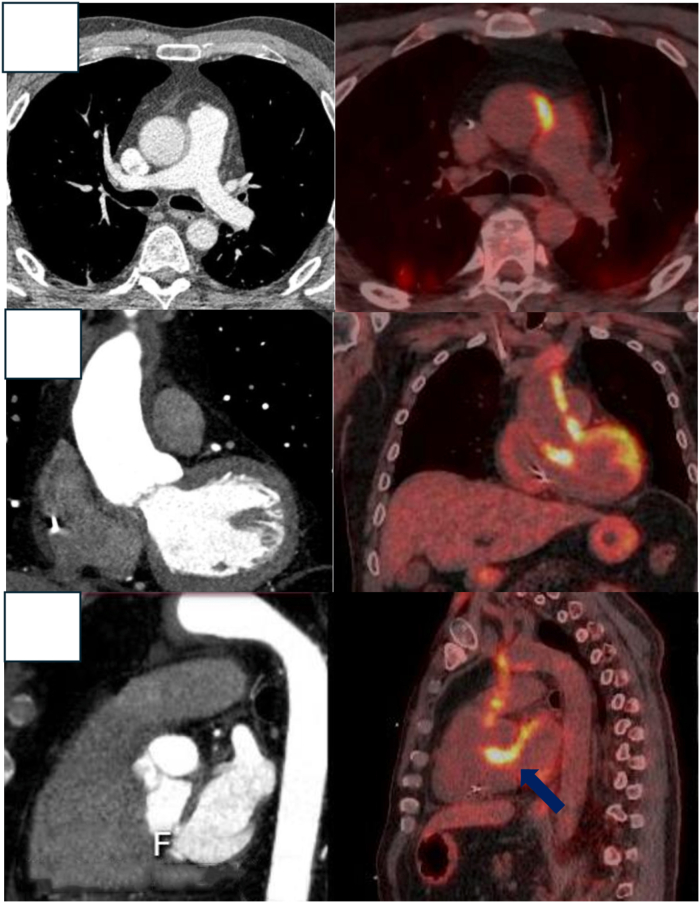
^18^F-FDG PET-CT Pathologic inflammatory uptake involving the wall of the ascending aorta, extending from its root to the proximal arch, with involvement of the aorto-mitral continuity (blue arrow). Transient pacemaker is also observed. ^18^F-FDG PET-CT = fluorine-18 fluorodeoxyglucose positron emission tomography-computed tomography.
